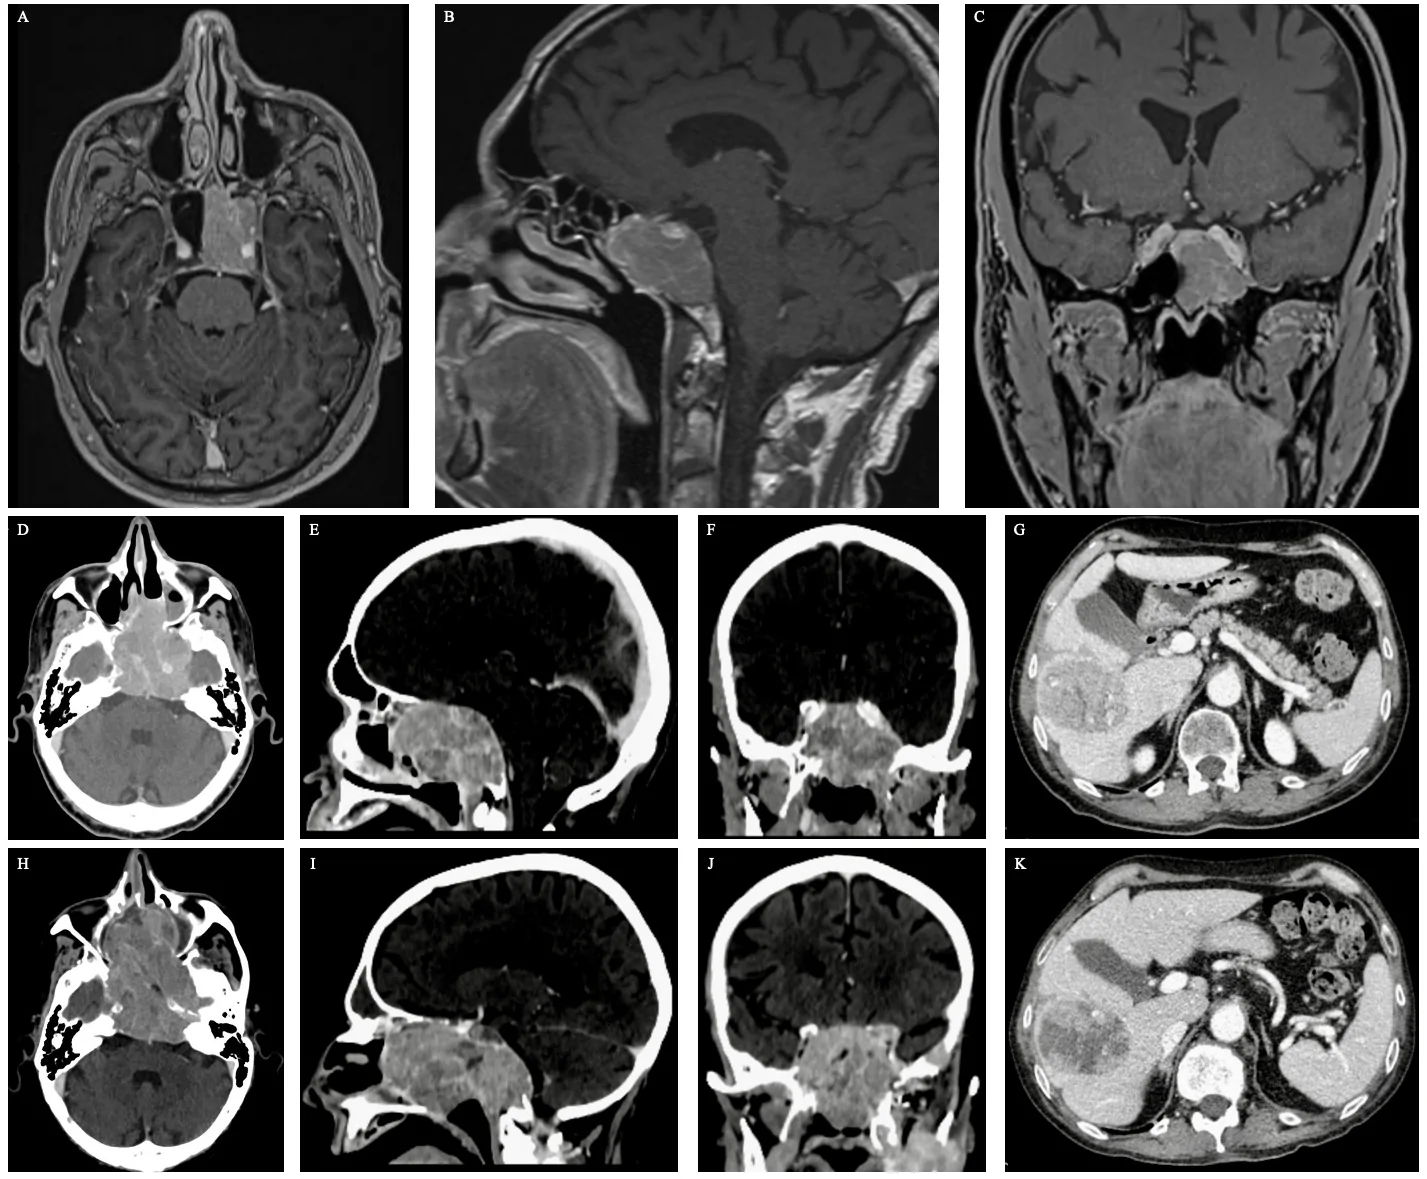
(A–C) Contrast-enhanced magnetic resonance imaging (MRI) of the maxillofacial region, obtained in June 2025, in axial (A), sagittal (B), and coronal (C) planes demonstrates an expansive mass in the median and left lateral portions of the sphenoid sinus. The lesion infiltrated the upper clivus (maximum dimensions: 42 × 22 mm) and the left cavernous sinus. (D–G) Contrast-enhanced computed tomography (CT) scans obtained in March 2026. Craniofacial CT images in axial (D), sagittal (E), and coronal (F) planes demonstrate a lesion in the sphenoid region (maximum axial dimensions: 81 × 55 mm). An axial abdominal CT image (G) demonstrates a lesion in hepatic segment VI measuring 78 × 59 mm. (H–K) Follow-up contrast-enhanced CT scans obtained in June 2026, after four treatment cycles of atezolizumab plus bevacizumab. Craniofacial CT images in axial (H), sagittal (I), and coronal (J) planes demonstrate progression of the sphenoid lesion (maximum axial dimensions: 86 × 60 mm). An axial abdominal CT image (K) demonstrates the lesion in hepatic segment VI, measuring 76 × 61 mm in maximum axial dimensions.
